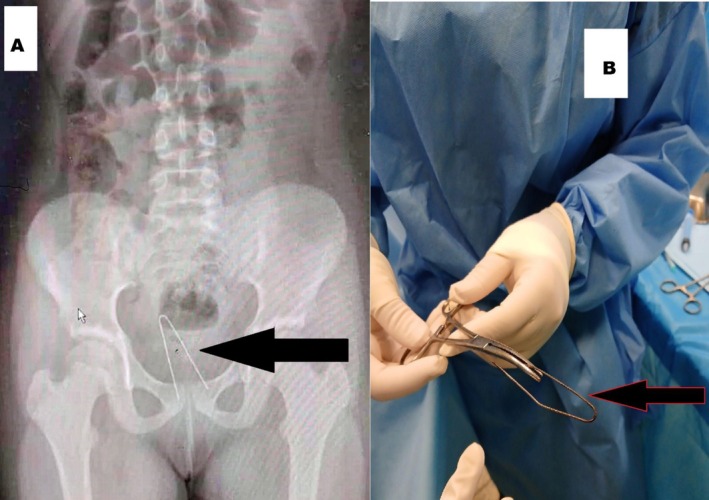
(A) Radiographic image demonstrating the presence of a U‐shaped hairpin located in the patient's mid‐pelvic region. (B) Showing the U‐shaped hairpin that was successfully extracted by the surgical team.
